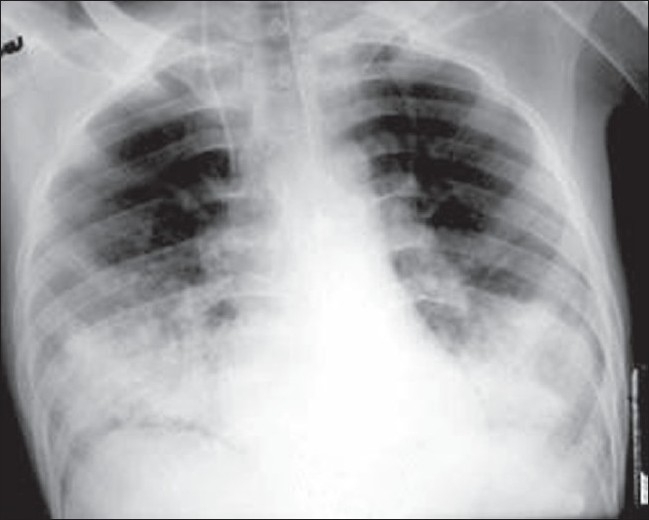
AP radiograph of the chest showing bilateral basal air space–filling lesions (consolidation)
